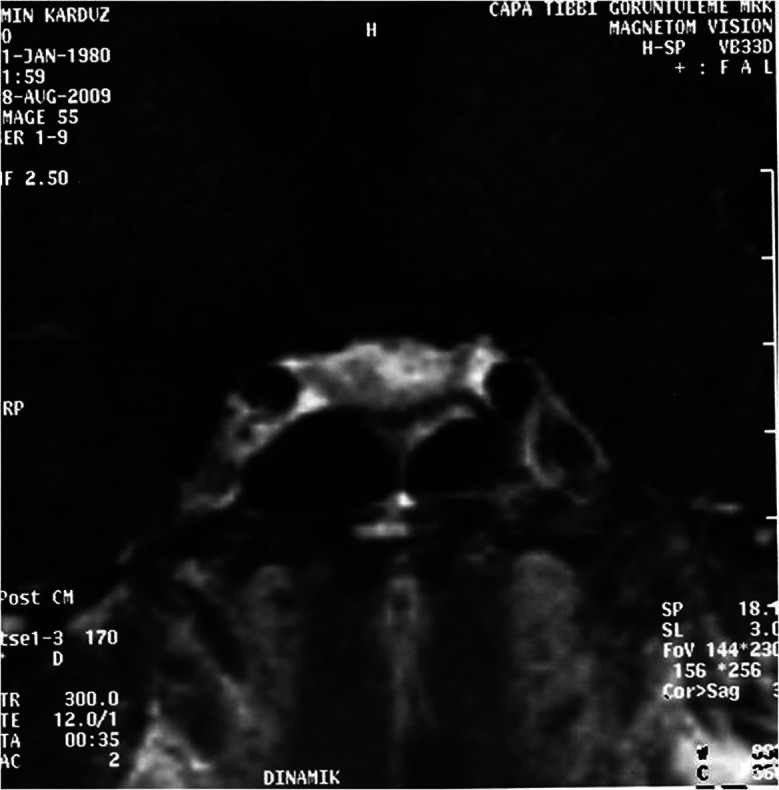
MRI of the sellar region after the second operation, showing postoperative changes.
